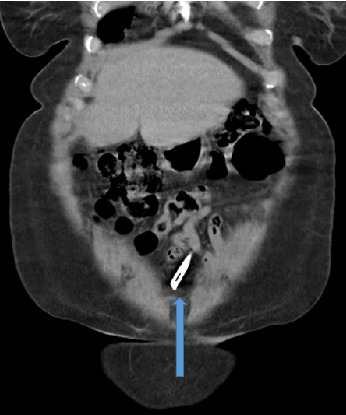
CT scan in coronal view revealed a surgical blade in the abdomen.
